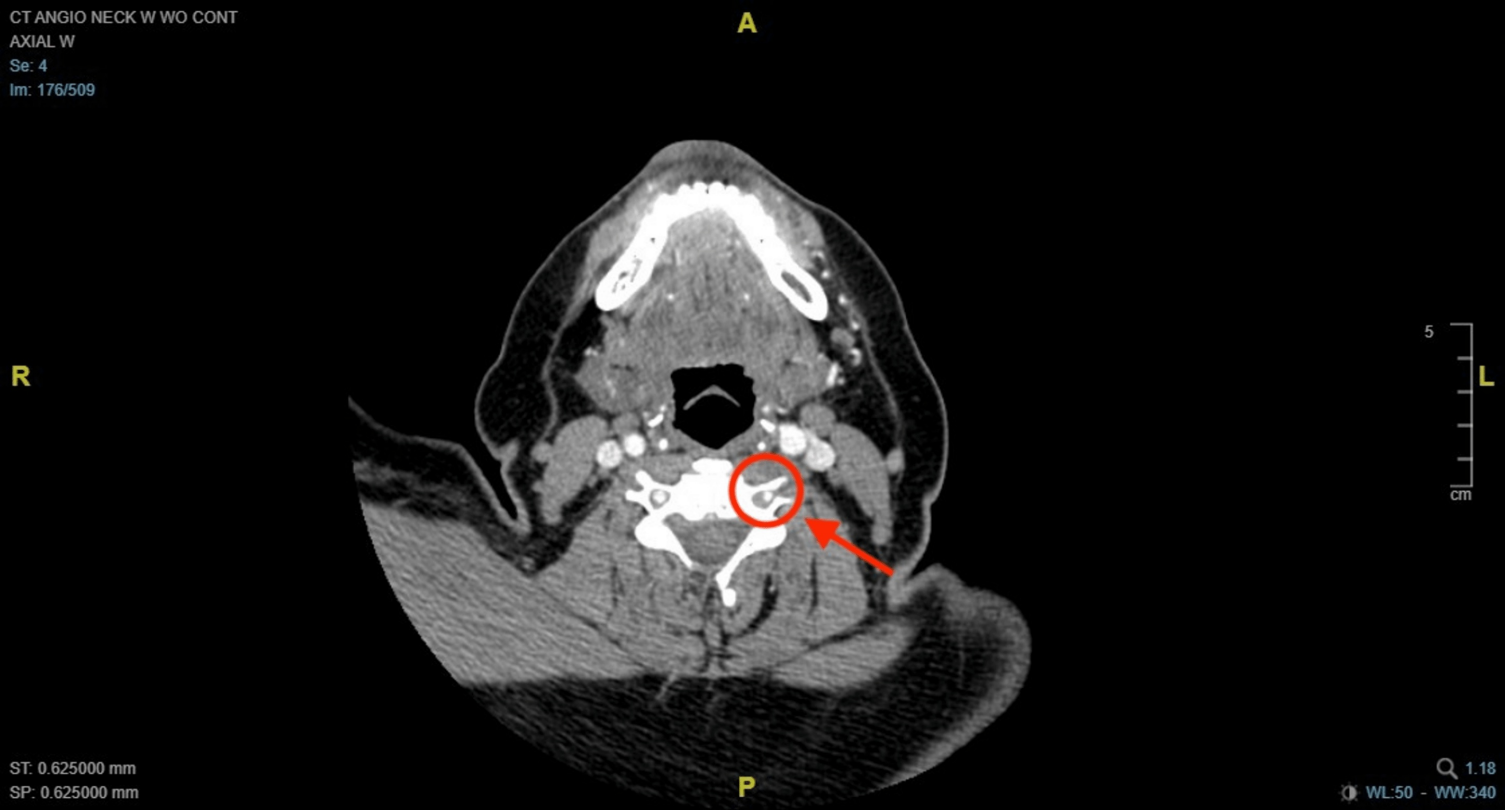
(Axial view) Computerized tomography angiography (CTA) of the neck with and without contrast showing peripheral hypoattenuation and luminal narrowing of the left vertebral artery, compared to the right side (red circle and arrow).
